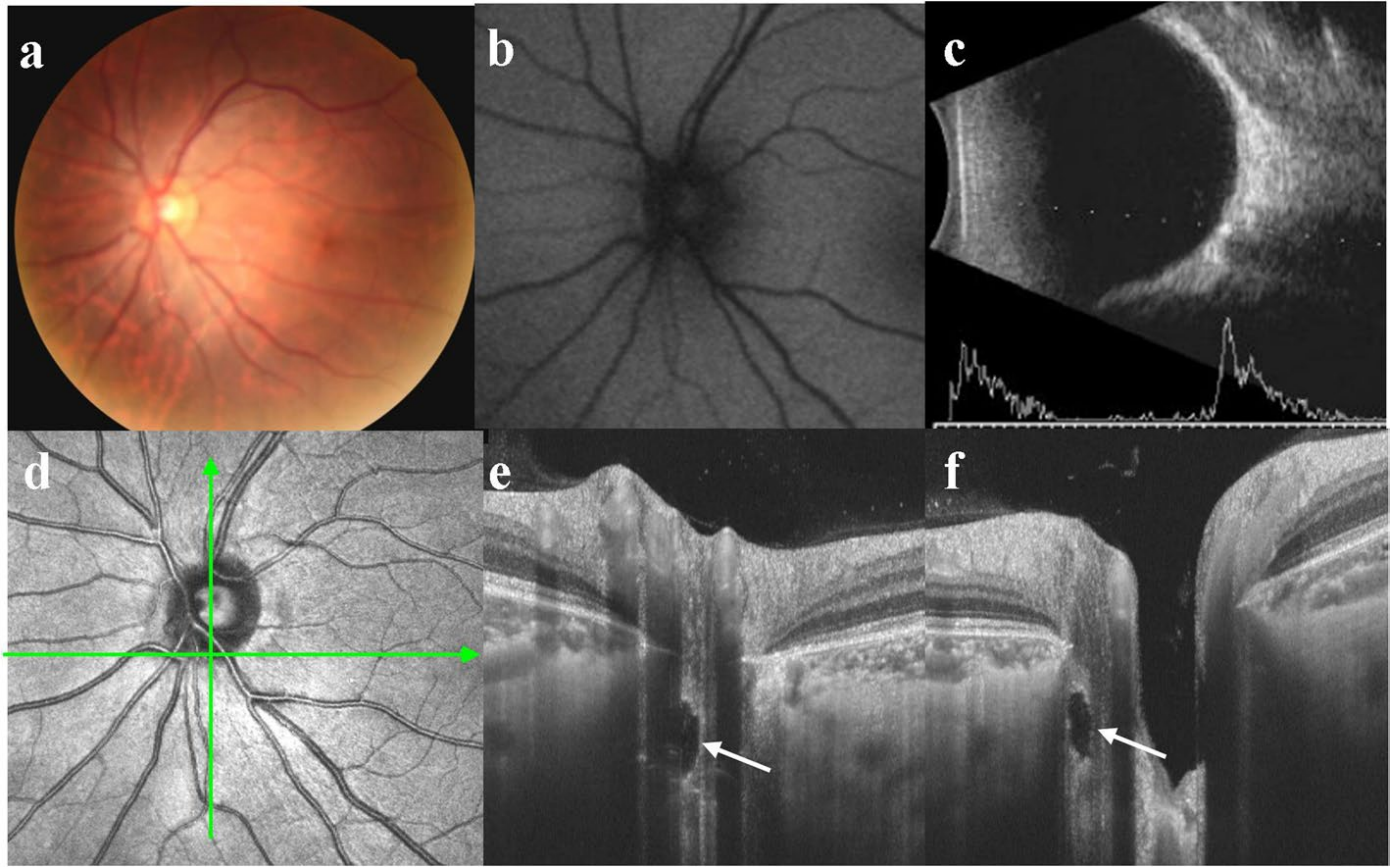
Multimodal imaging of a typical buried ODD a There was no noticeable pseudoedema in the optic disc. b No autofluorescence was detected. c B-scan ultrasonography showed strong echo spots with acoustic shadowing. d Horizontal scan line corresponded to e, vertical scan line corresponded to f. e/f An ODD was located near the rim of the optic disc (white arrow), and there was no highly reflective mass around the optic papilla
